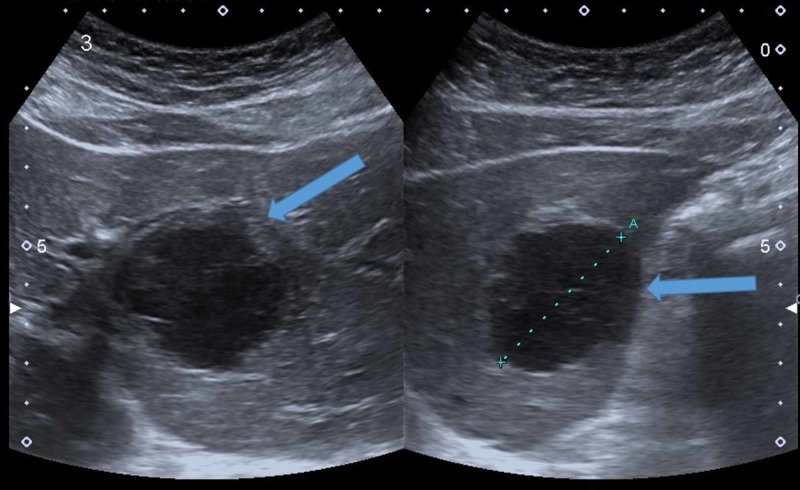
Abdominal US discovered two hypoechogenic changes in the liver (arrows). US, ultrasound
